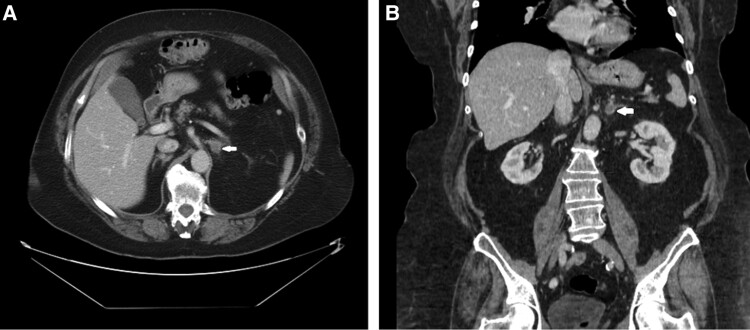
(A) CT scan axial showing a left adrenal mass as identified by the white arrow. (B) CT scan coronal view showing a left adrenal mass as identified by the white arrow.
